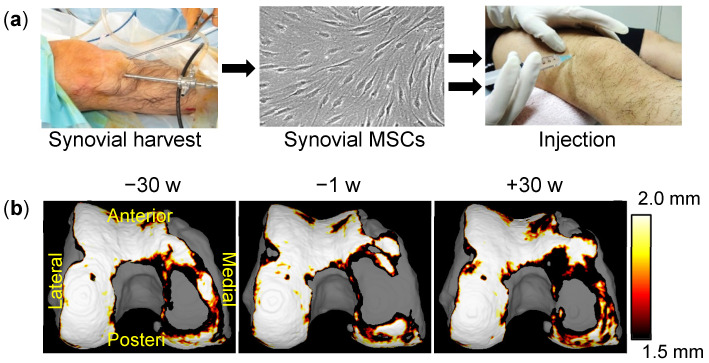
Clinical study of synovial MSC injections into OA knees. (a) Scheme of a clinical study of autologous synovial MSC injections into the OA knees of human patients. The synovium was harvested and enzymatically digested. The resulting synovial MSCs were expanded with autologous human serum and then injected into the knee twice at a 15-week interval. (b) Cartilage thickness mappings in a representative patient who underwent synovial MSC injections into a knee at time 0 and 15 weeks. MRI examinations were performed at −30, −1, and +30 weeks. Using the software we developed, the cartilage area was automatically extracted and visualized in three dimensions. Femoral cartilage at the posteromedial region at −30 weeks had decreased at −1 week but increased again at +30 weeks.
